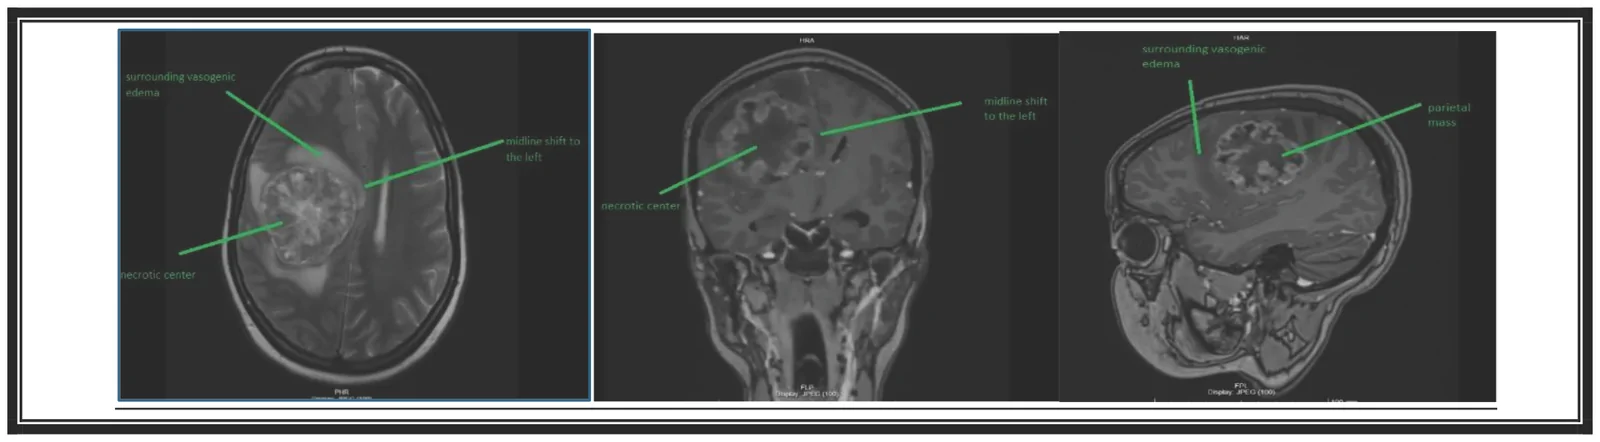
MRI: (A) axial T2 imaging, (B) coronal T1 with contrast imaging, and (C) sagittal T1 with contrast showing a heterogeneously enhancing mass with surrounding edema causing midline shift to the left.
